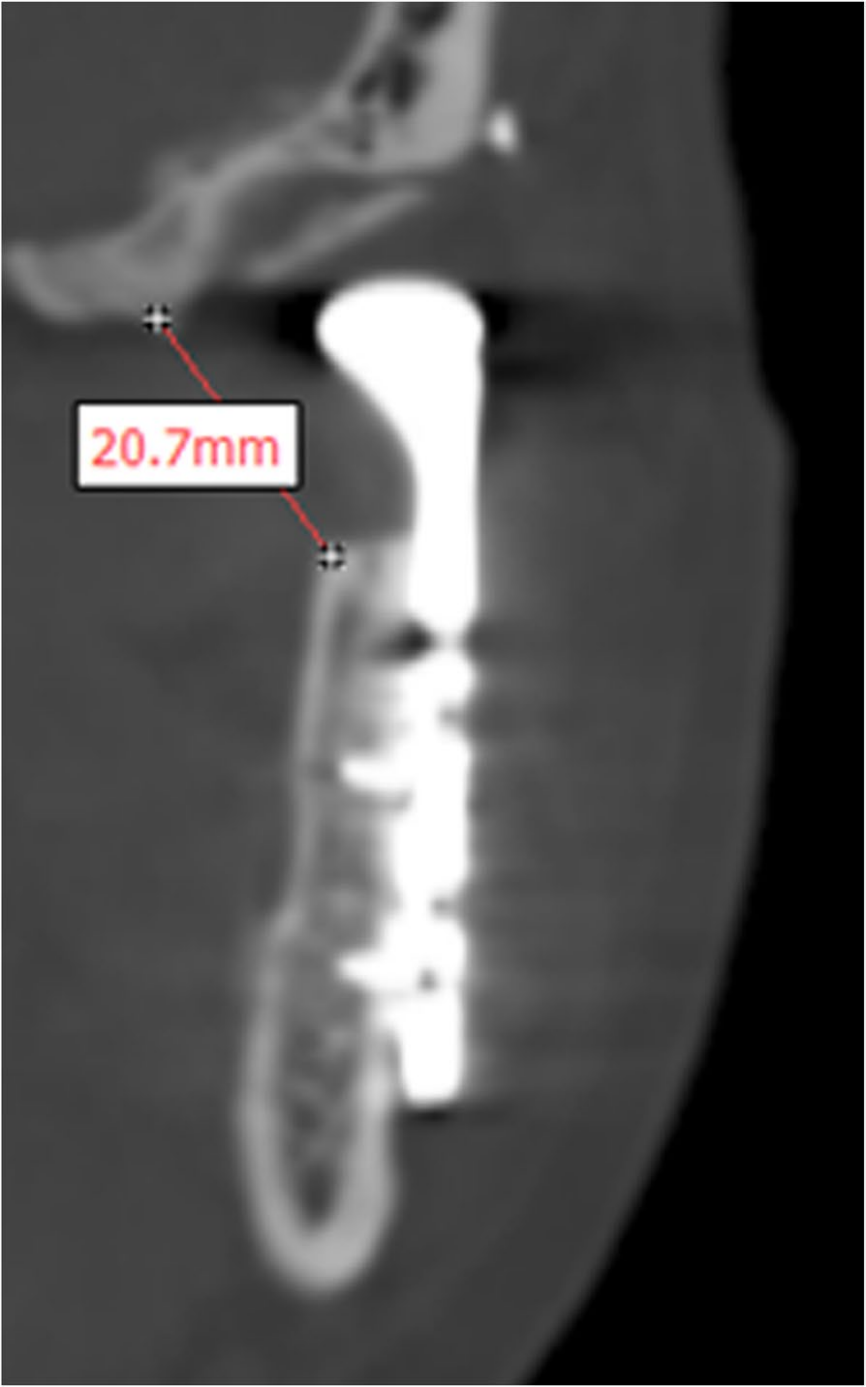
Joint space measurement in the CT coronal reconstruction 1 week after operation
